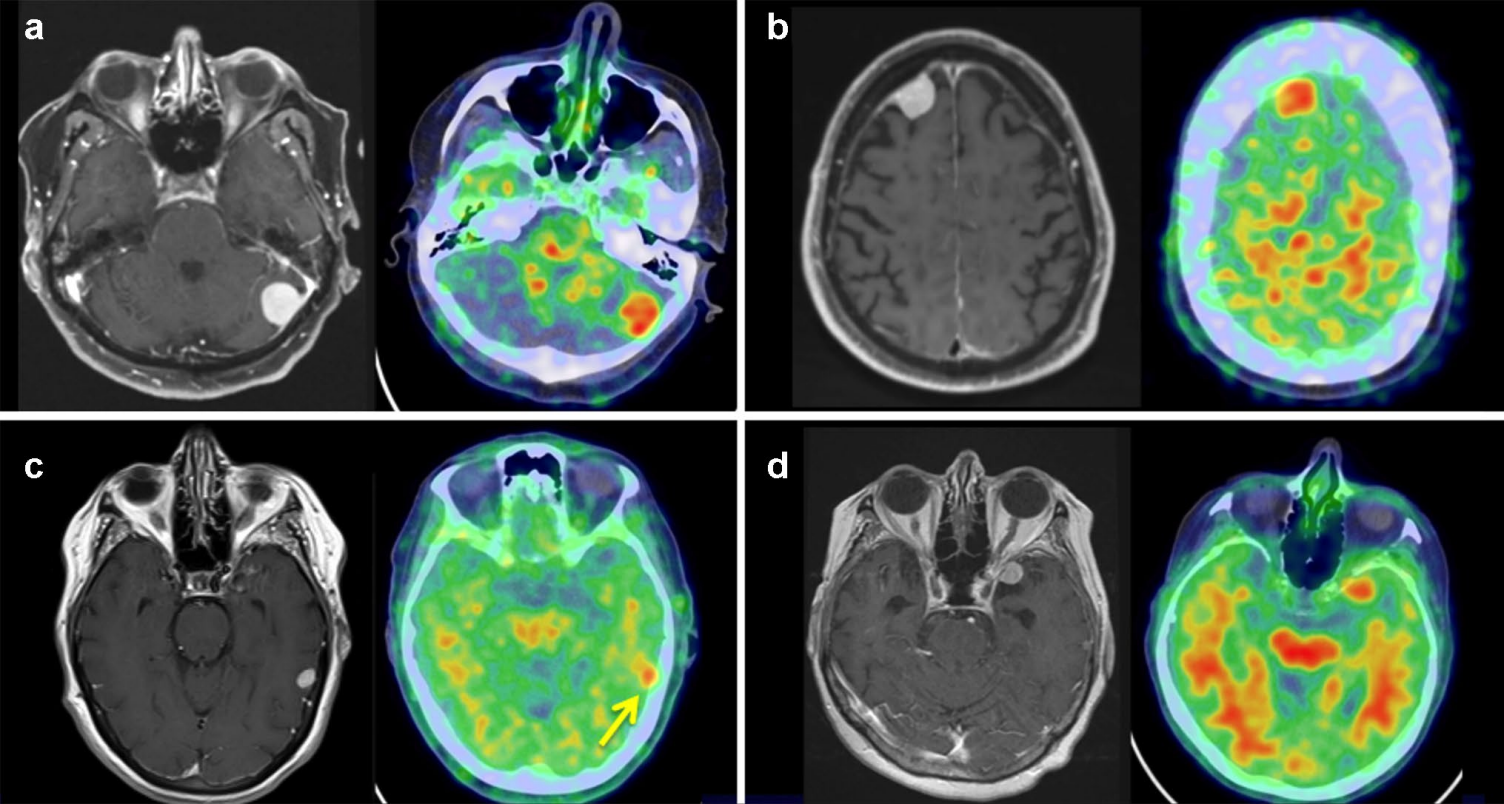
Additional meningioma PiB PET/CT images demonstrating PiB uptake in tumors abutting the left cerebellar hemisphere (a), right frontal lobe (b), posterior left temporal lobe (c), and left anteromedial temporal lobe (d), with companion T1-weighted post-contrast MR images
